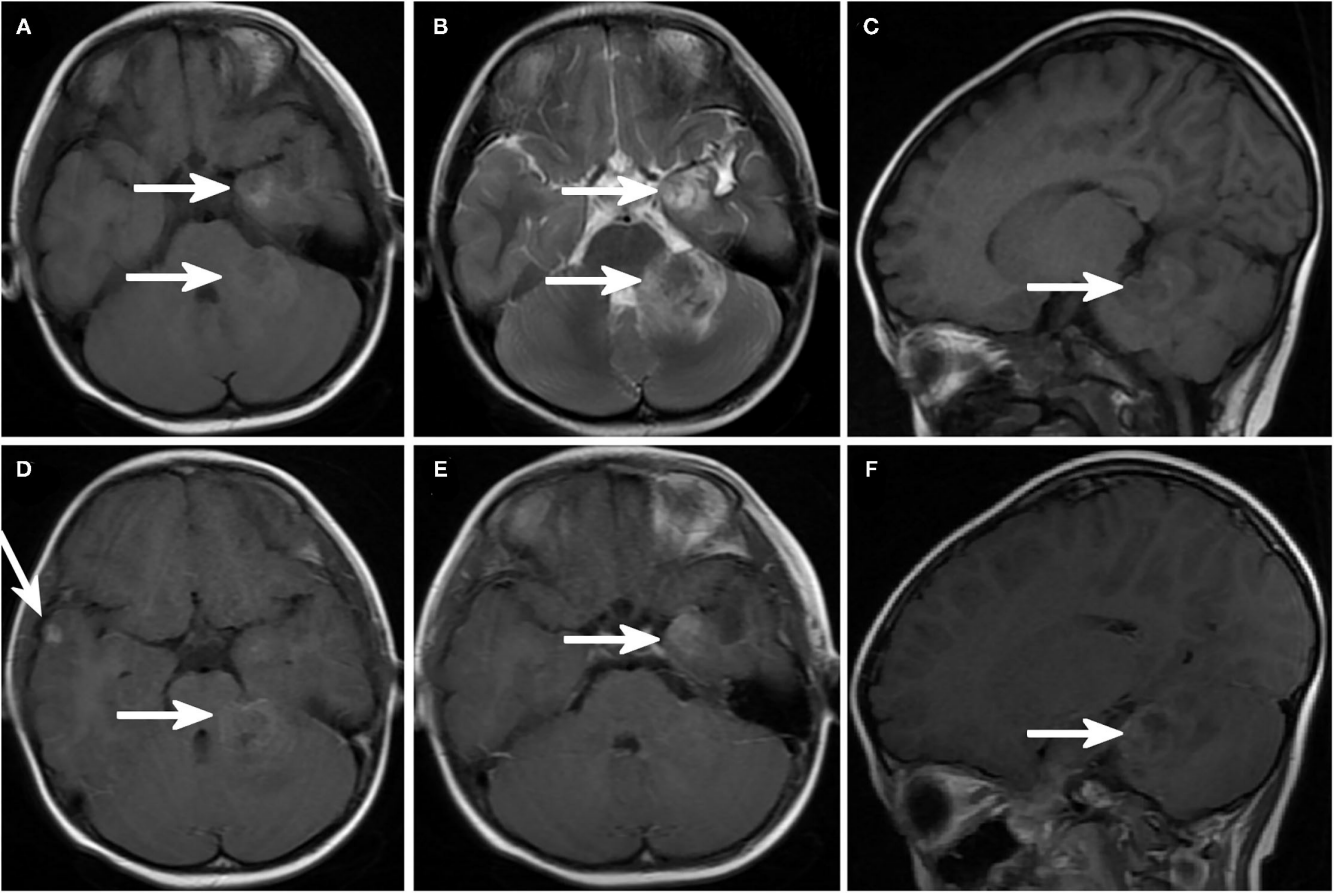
Brain magnetic resonance imaging (MRI) showing intracranial metastatic lesions. (A) Axial T1-weighted image and (B) axial T2-weighted image demonstrated a lesion in the left temporal lobe and left cerebellar hemisphere with prominent cerebral edema around the lesion. Sagittal T1-weighted image (C) showed a cerebellar lesion. (D–F) Enhanced MRI sagittal T1-weighted image and (D) right temporal lobe and left cerebellar hemisphere lesions. (E) Enhanced sagittal MRI image showed left cerebellar hemisphere lesions.
